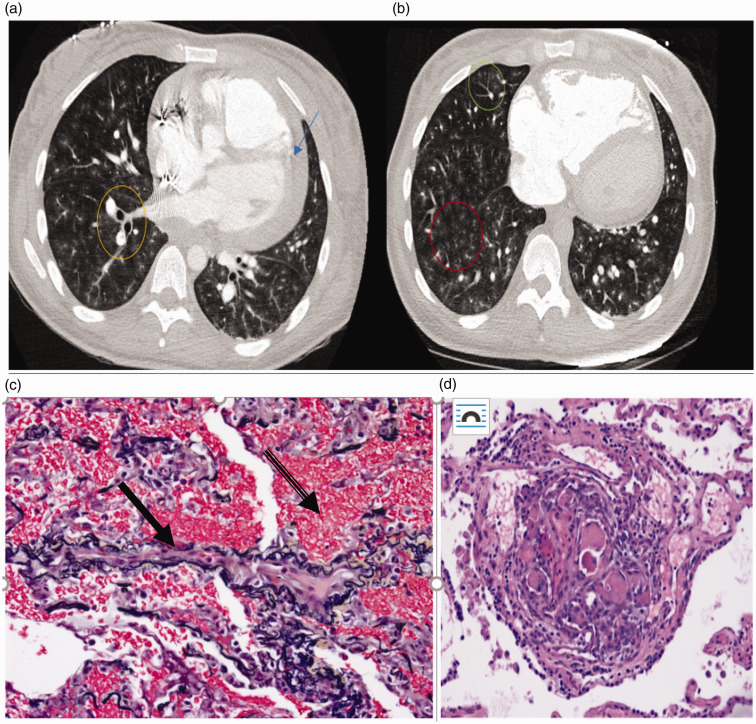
(a and b) Case 2: Axial images from computed tomography angiogram
